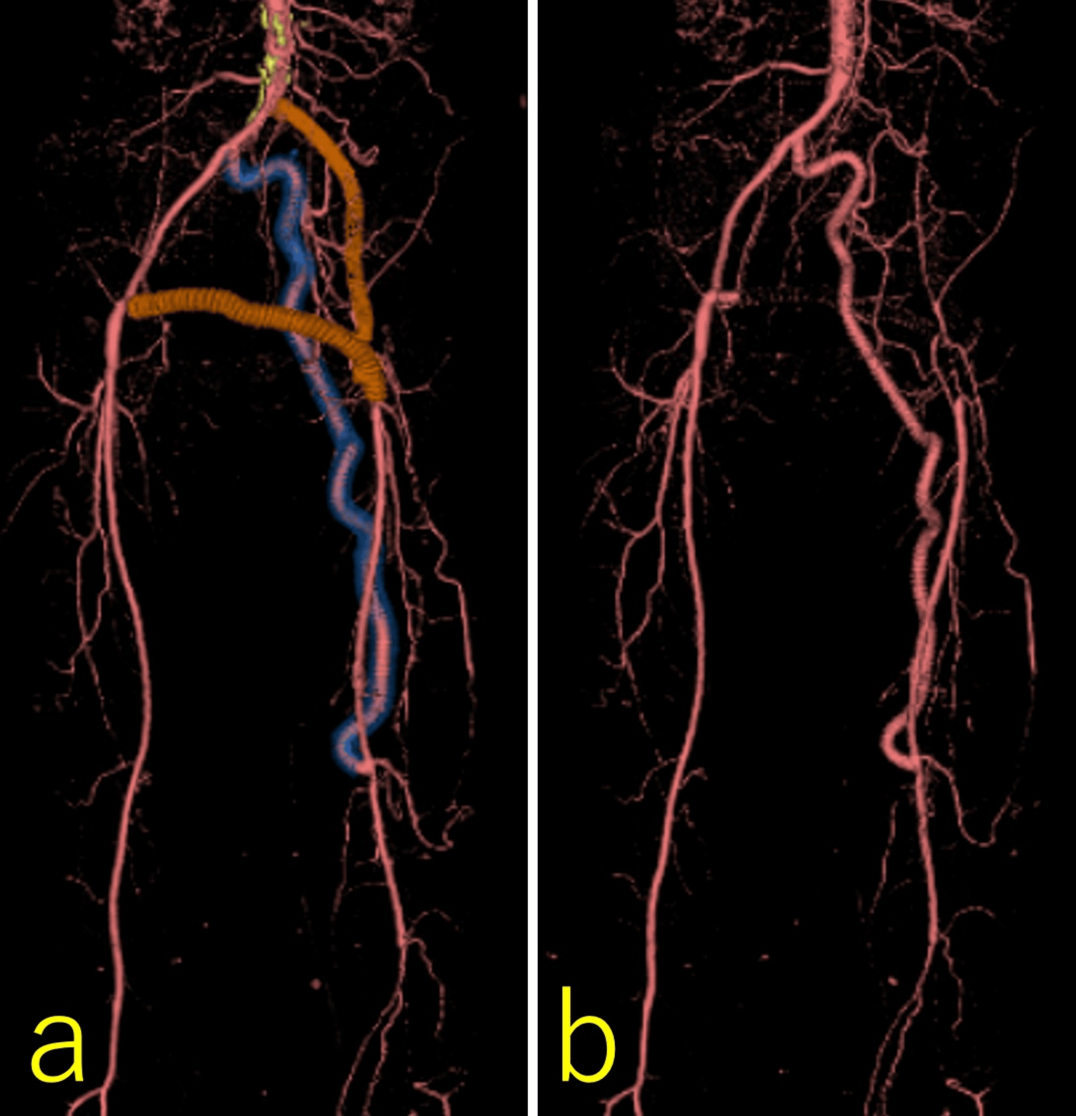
Postoperative findings of obturator foramen crossover bypass grafting and thrombectomy a: contrast-enhanced three-dimensional CT showing the previously implanted grafts, demonstrating good patency of the obturator foramen crossover bypass; b: contrast-enhanced three-dimensional CT without visualization of the previously implanted grafts.
